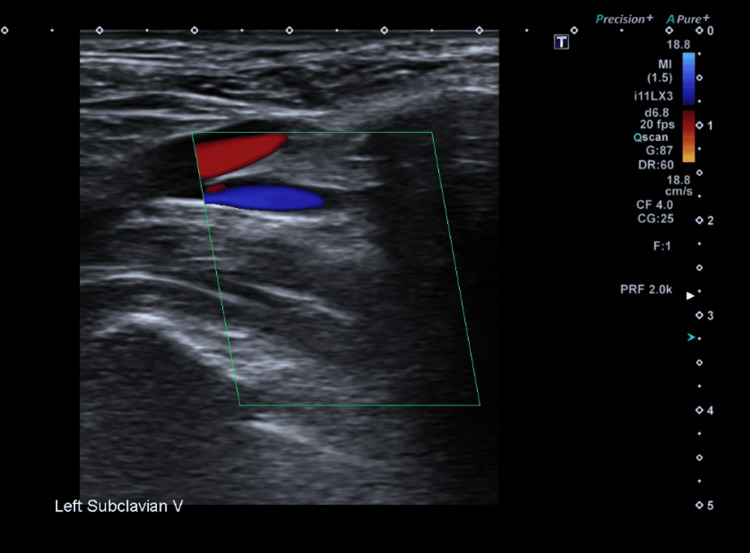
US shows reduced colour Doppler flow in the left subclavian vein (green box) US: ultrasound
